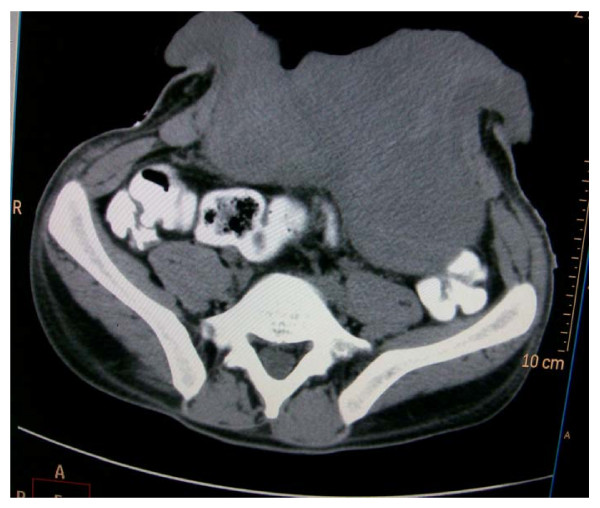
CT scan of the abdomen showing the anterior abdominal wall tumor.
